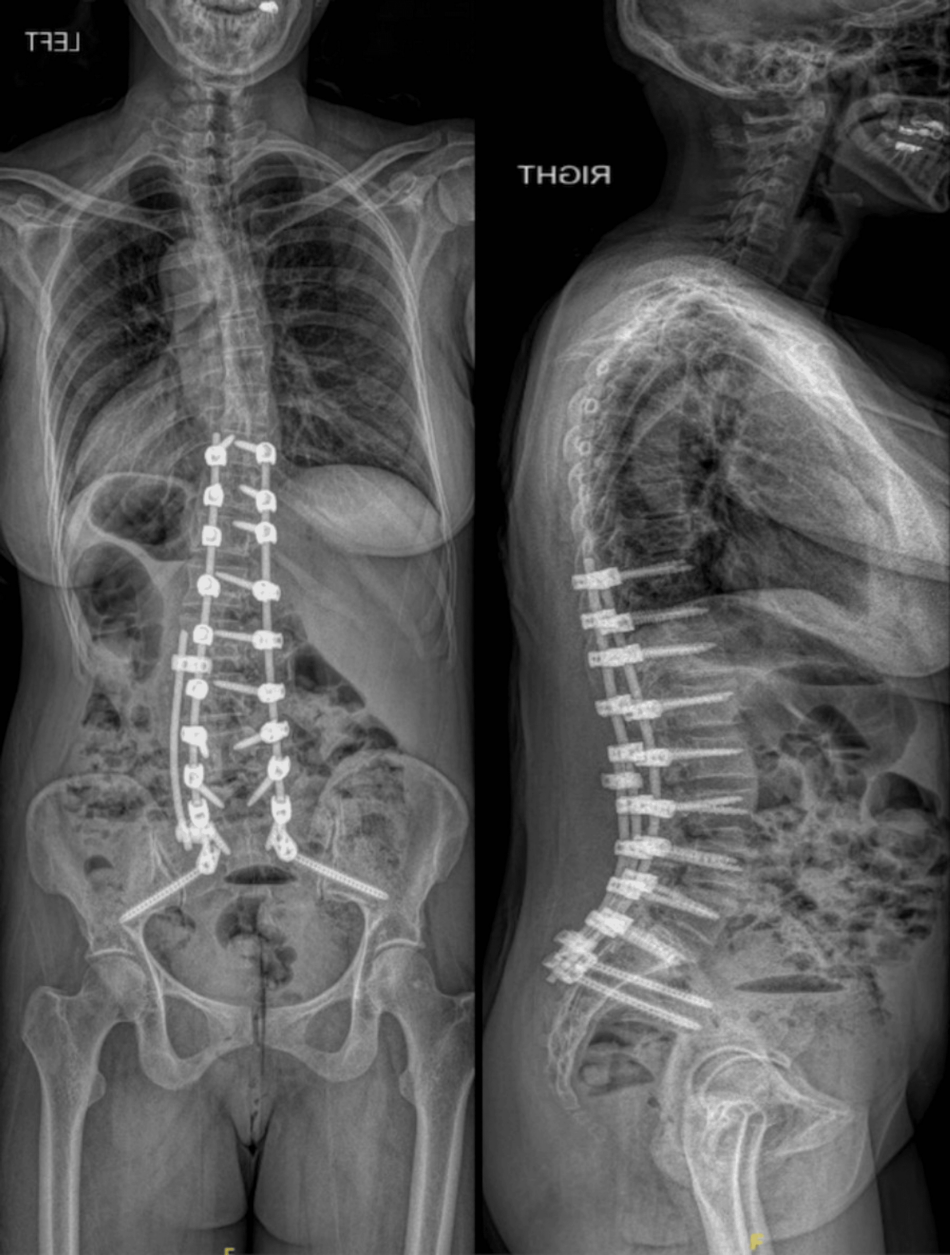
Postoperative anteroposterior (left) and lateral (right) long-cassette radiographs demonstrating T10-ilium posterior instrumentation, correction of the preoperative coronal deformity, and maintenance of global sagittal alignment.
